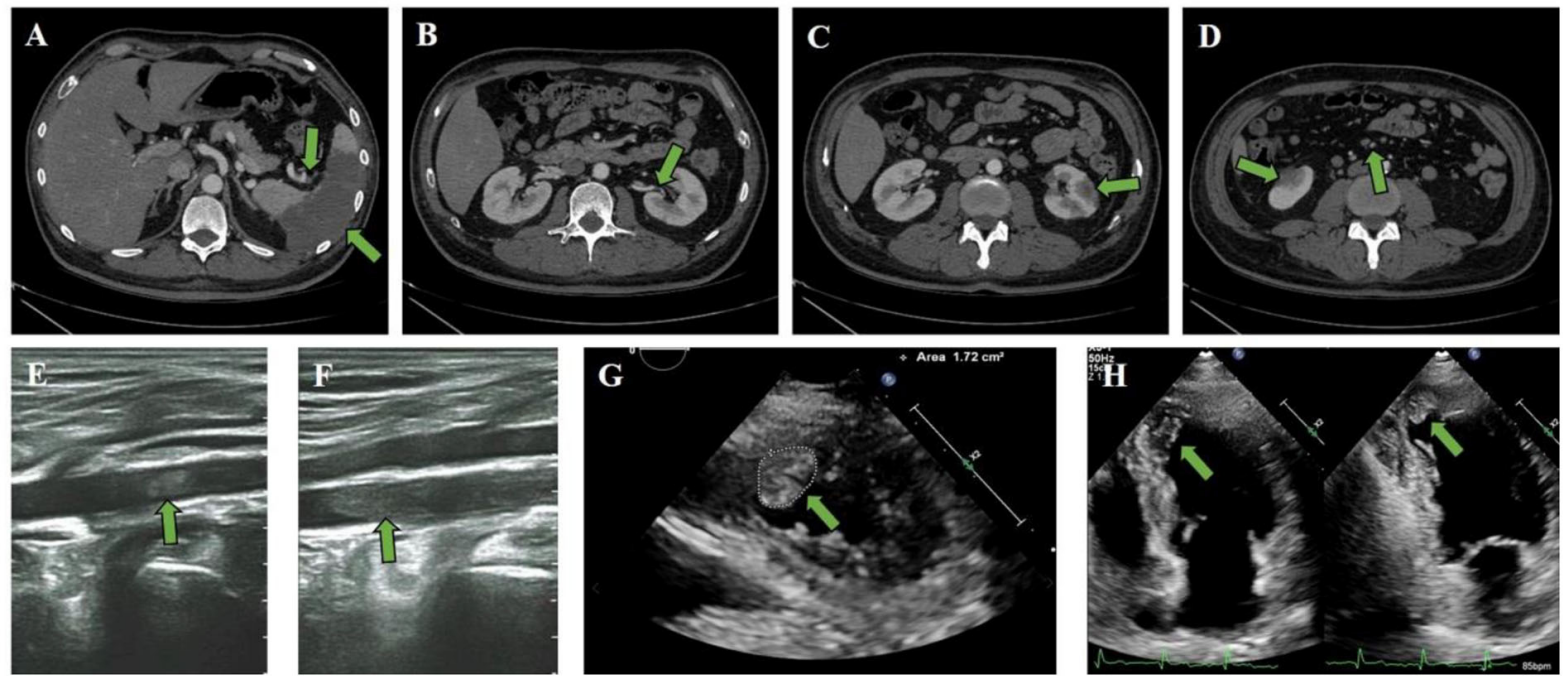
Clinical CT scan and ultrasound imaging. (A–F) Enhanced CT scan revealed (A) splenic infarction and splenic artery thrombosis, as well as (B) left renal artery embolization, (C) left renal infarction, and (D) superior mesenteric artery thrombosis and right renal infarction. Ultrasonography of the lower limb artery revealed (E) right popliteal artery thrombosis and (F) left posterior tibial artery thrombosis. (G,H) The attached thrombus (size 1.13 × 1.77 cm and area 1.72 cm^2^) was observed in the apex part of the LV.
